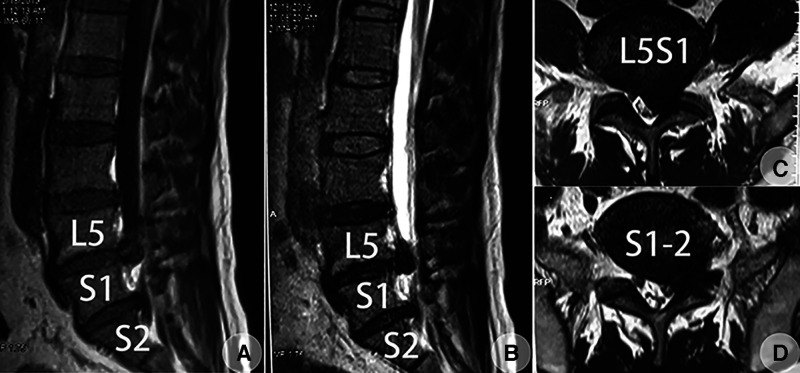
MRI of the lumbar spine taken on 22 September 2019, revealed huge LDHs at the lowest two free levels at the left side, which was later confirmed to be L5S1 and lumbarized S1-2 disc [type IV TD (lumbosacral transitional disc) according to Wigh et al.’s (4) classification based on the following whole spinal images]. (A) Sagittal plane through the left lateral recess (T1WI). (B) Sagittal plane through the left lateral recess (T2WI). (C) Transverse plane through the L5S1 disc (T2WI) showed an axillary type LDH. (D) Transverse plane through the well-developed S1-2 disc (T2WI) showed a shoulder type LDH.
